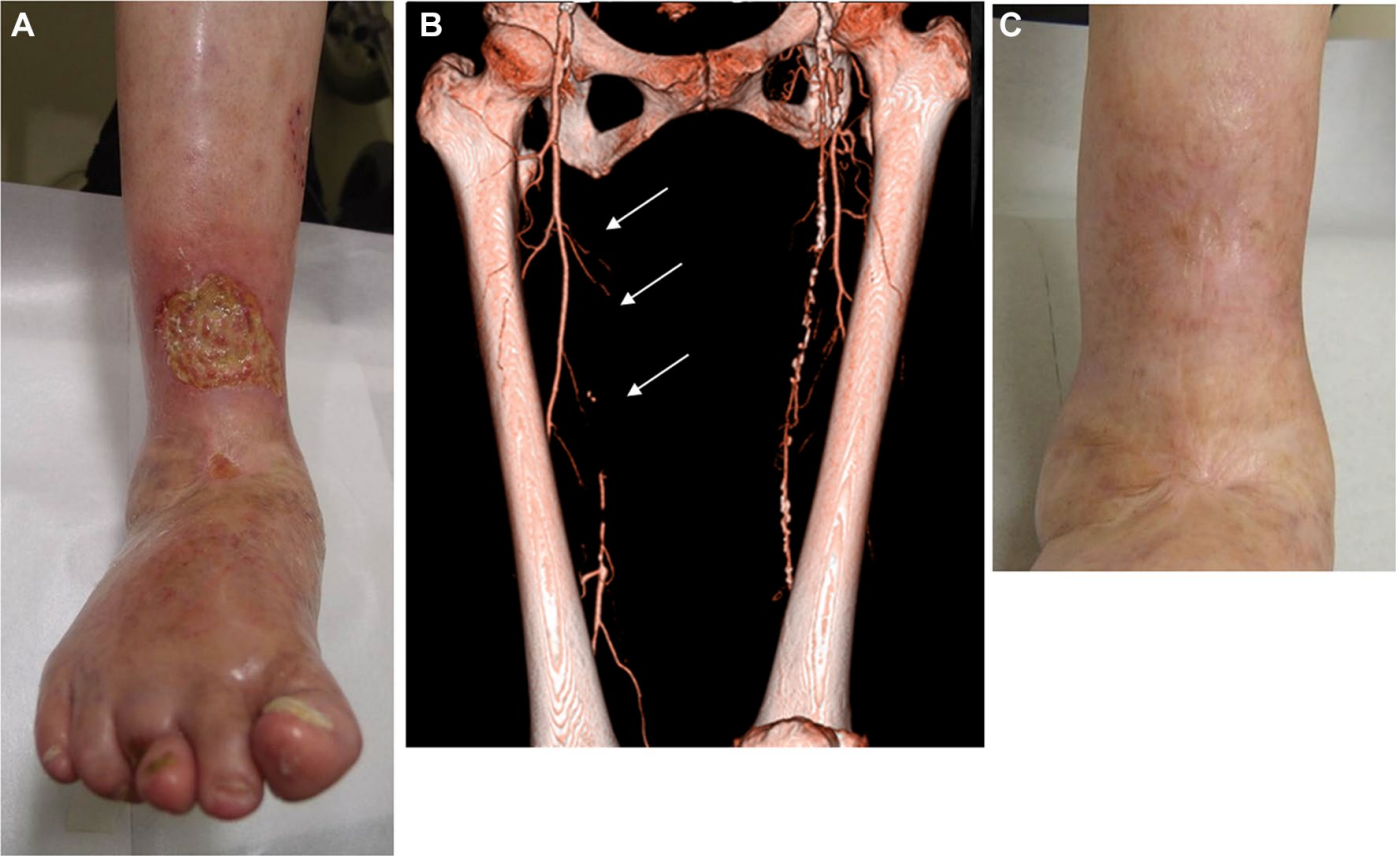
Venous stasis ulcer complicated by peripheral artery disease. a A 74-year-old female presenting with ulcer above the ankle. b Enhanced CT revealed long occlusion in the femoropopliteal artery (arrows). However, SPP around the ankle was almost 40 mmHg, suggesting preservation of microcirculation. c Wound management including compressive bandage facilitated complete wound healing 6 months later without revascularization
